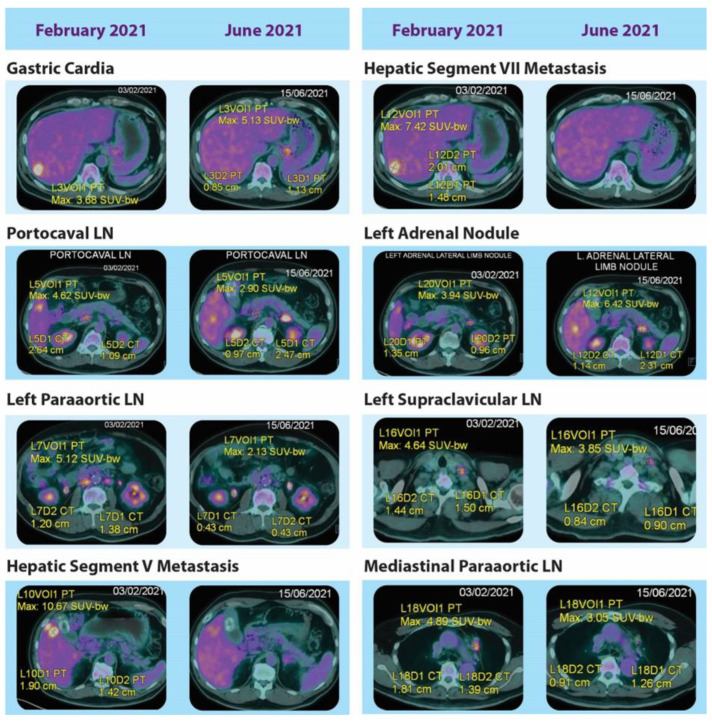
PET/CT images before and after 3 cycles of trastuzumab deruxtecan, showing varied responses in the primary and metastatic sites.
